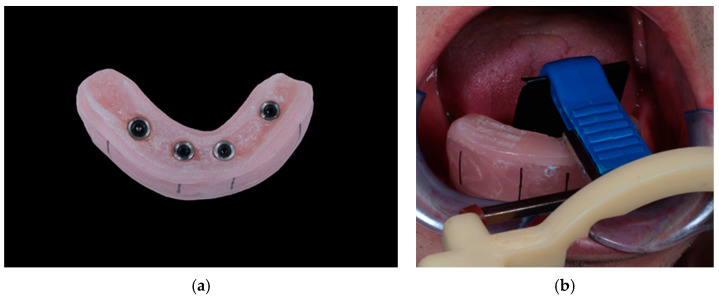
View of a radiological splint: (a) basal view of a radiological splint, including MH2 matrices and O-rings, and (b) clinical set-up for standardized radiograph recording.
